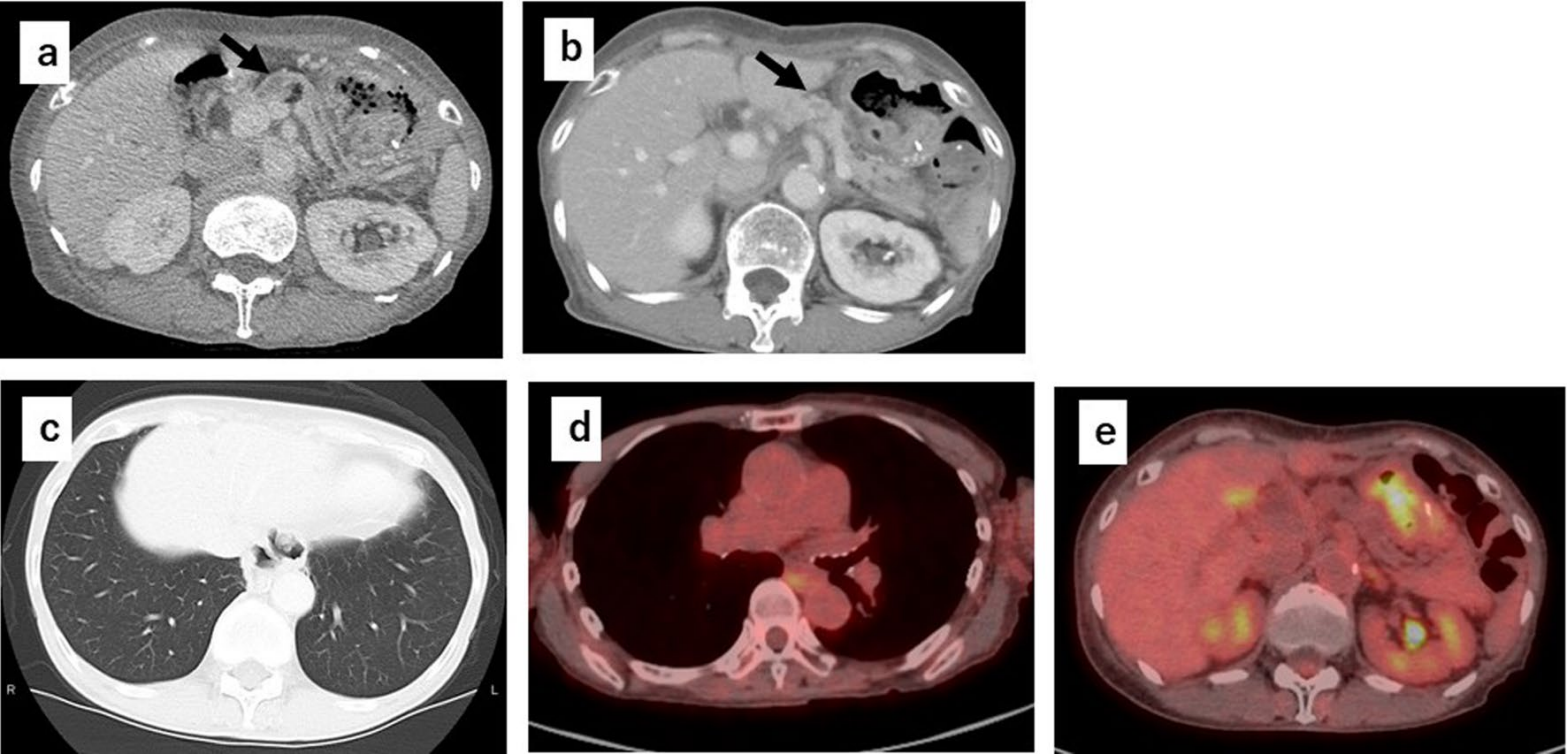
CT images of the abdominal area. a Abdominal CT showing unstained nodule in the pancreas (black arrow) and dilation of the caudal pancreatic duct. b CT indicating the obscured nodule in the pancreas (black arrow) after radiotherapy. c CT scan showing absence of metastatic lesions and subpleural and pleural effusion after radiotherapy. d, e PET–CT scan showing the absence of abnormal accumulation in the lung area and pancreas
